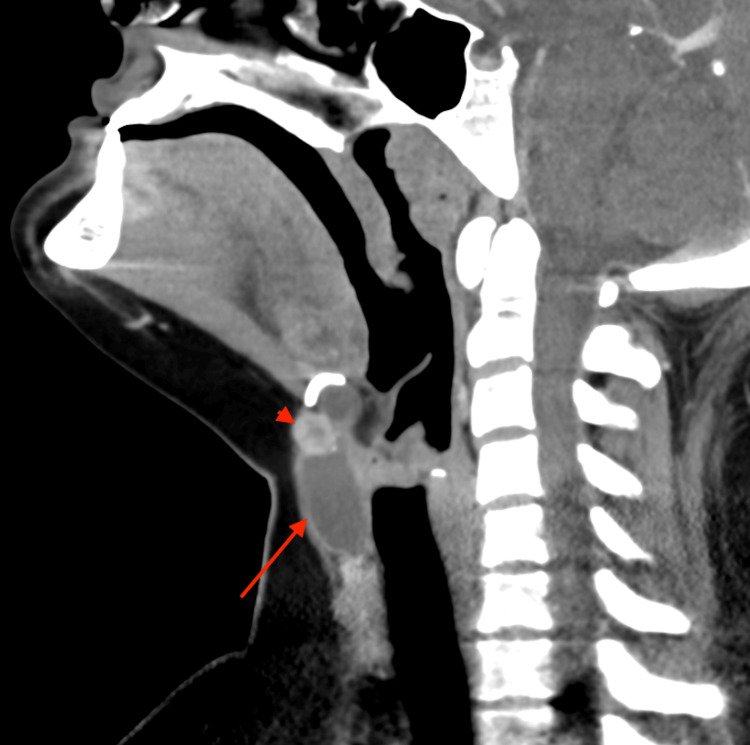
Sagittal CT of the neck with contrast. Sagittal CT revealed a cystic tubular structure (red arrow). There was a peripherally hyperattenuating and centrally hypoattenuating lesion (red arrowhead) in the superior midline aspect of the cyst with multiple adjacent punctate calcifications, measuring 1.0 x 1.2 x 1.3 cm.
